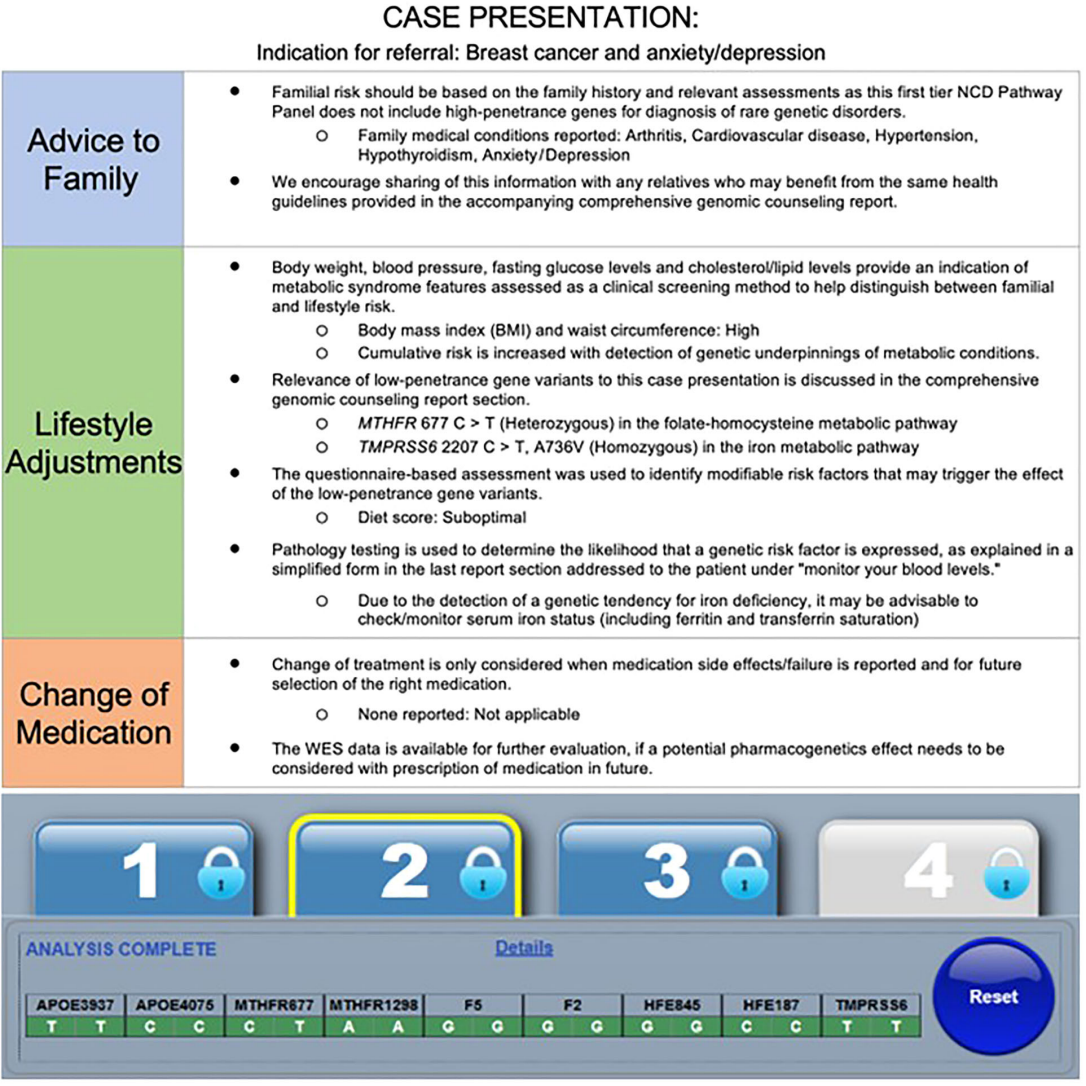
Excerpt from a pathology‐supported genetic testing report of multiple noncommunicable disease (NCD) pathways influenced by familial risk and environmental factors. Top: Multidisciplinary risk stratification summary extracted from the comprehensive genomic counseling and health monitoring sections of the NCD point‐of‐care assay extended to whole exome sequencing (WES). Bottom: Detection of two low‐penetrance gene variants associated with homocysteine accumulation (*MTHFR* 677 CT heterozygote) and a genetic tendency for iron deficiency (*TMPRSS6* 2207 TT homozygote), respectively.
